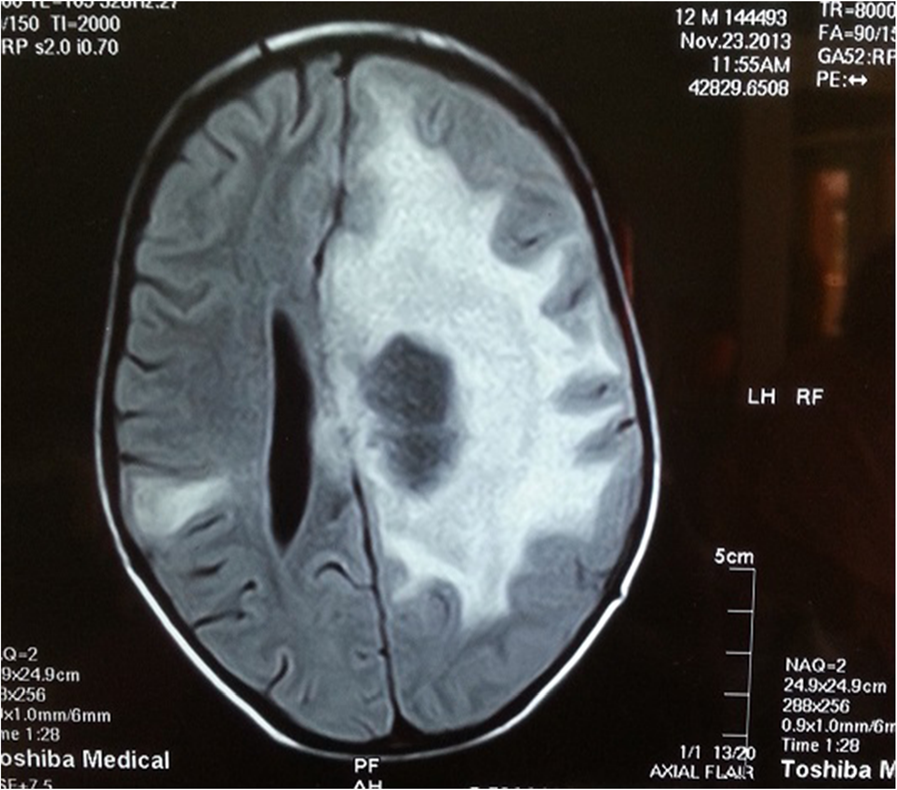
Magnetic resonance imaging of the brain showing numerous hemorrhagic mass lesions in the left temporal, left frontoparietal, and right parietal lobes
